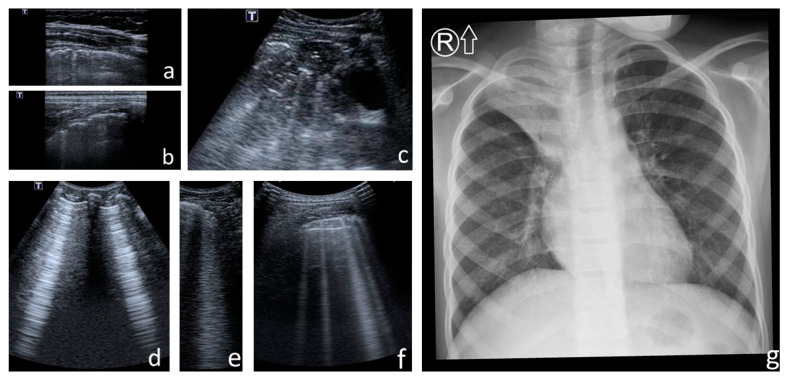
Exemplary collation of lung ultrasound (LUS) and chest X-ray (CXR) images. (a–f) LUS—elements assessed with the CF LUS score: (a,b) pleural line abnormalities; (c) consolidation covering the entire upper lung field; front and rear, with heterogeneous echogenicity and irregular static air bronchogram/air trapping (hyperechoic points, spots, and linear structures within the consolidation)—cirrhosis; (d) Am-lines; (e) confluent B-line; (f) numerous B-lines. (g) CXR—elements assessed with the modified Chrispin–Norman score: barrel chest, excessive transparency of the lung fields (overinflation of the middle lobe and left upper lobe), cirrhosis of the upper right lobe with atelectasis and air bronchogram, bilateral lesions consistent with bronchiectasis with wall thickening (linear peribronchial opacities including tram-track sign, and ring shadows). Total number of points on the CF LUS score—29, on the modified Chrispin–Norman score—17.
